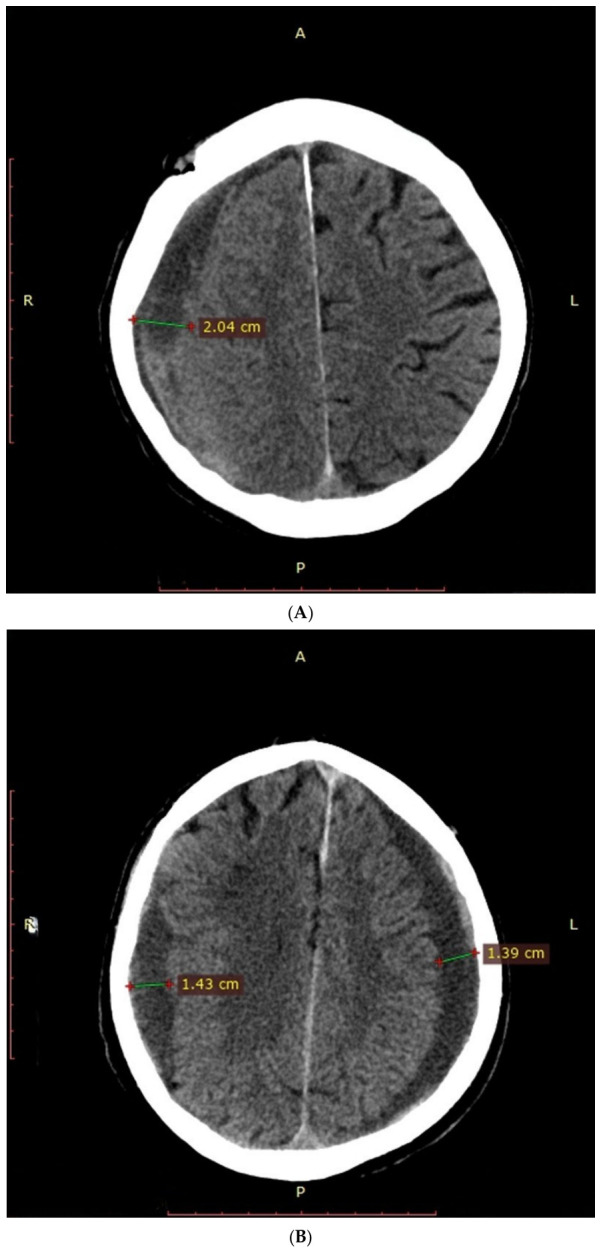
(A) Axial section of the brain on non-contrast CT (radiological image of a right-sided subdural hematoma causing marked compression of the brain tissue—measurement of the hematoma width). (B) Axial section of the brain on non-contrast CT (radiological image of bilateral subdural hematomas causing marked compression of the brain tissue on both sides—measurement of the hematoma widths). Own material. A—Anterior; L—Left; P—Posterior; R—Right.
